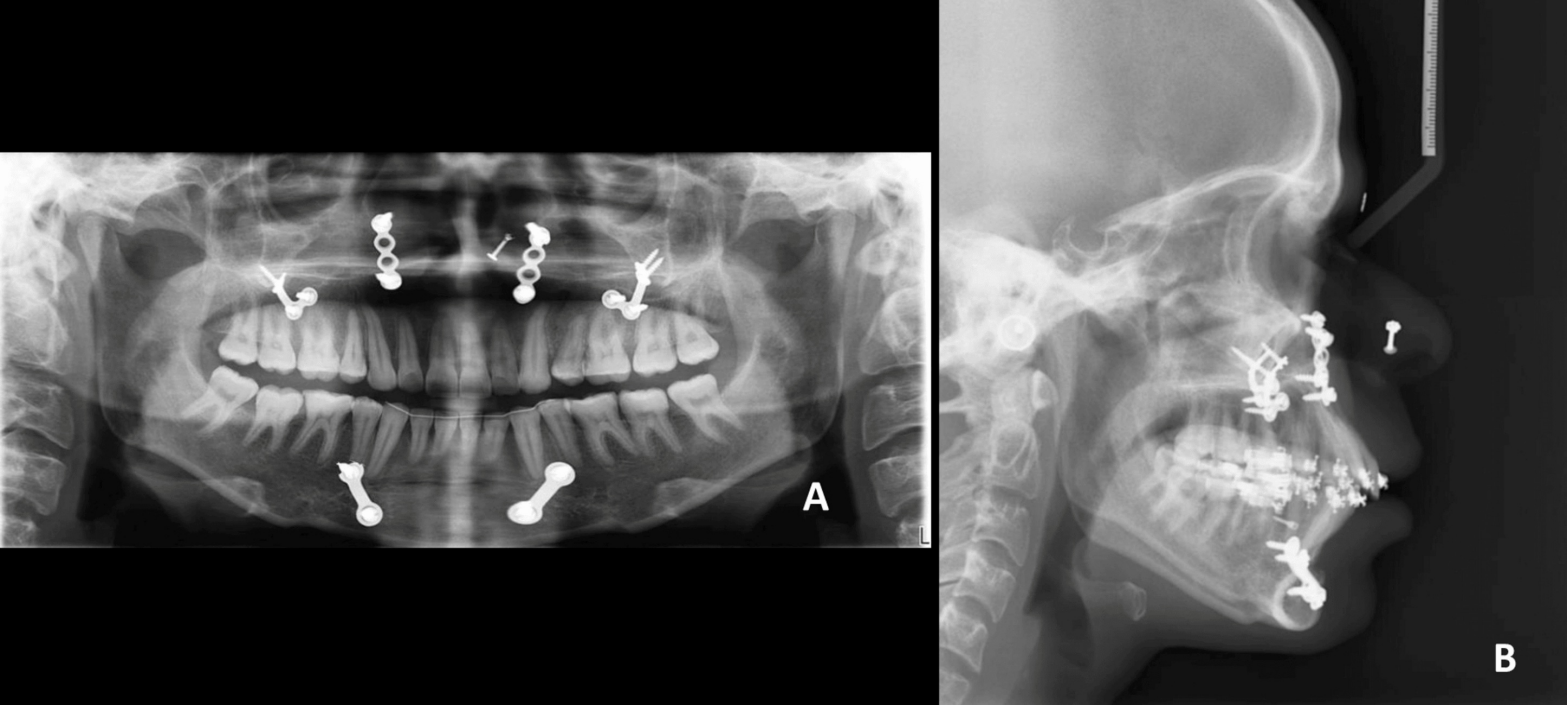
Post-treatment radiographs. A: Orthopantomogram; B: Lateral cephalogram
